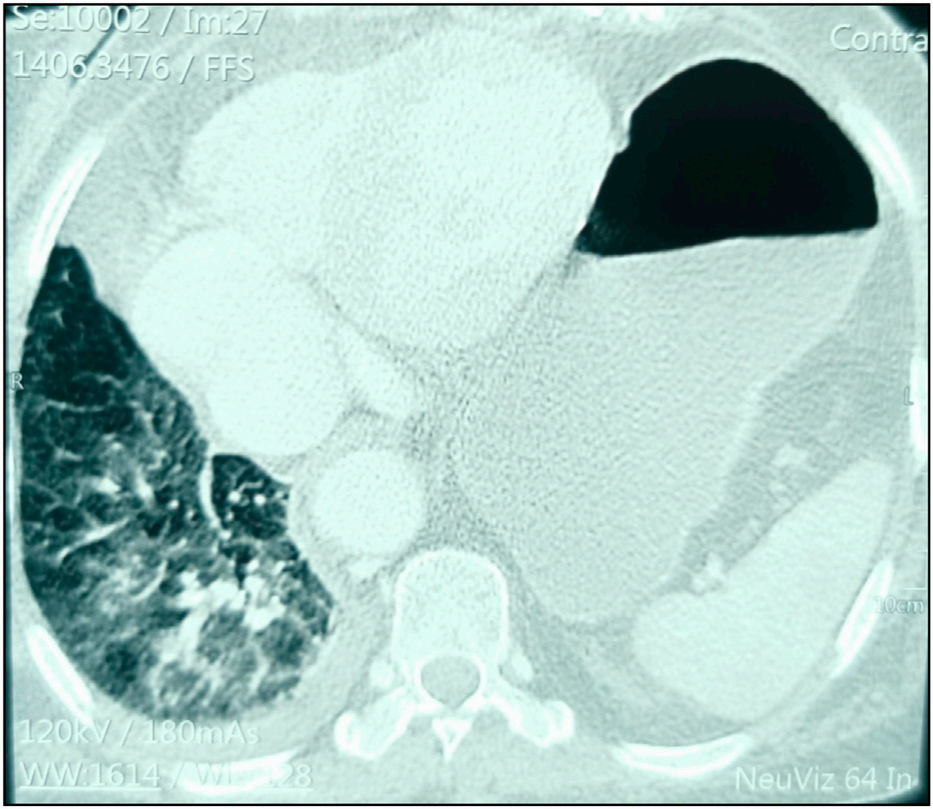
Chest computed tomography showing the spleen and stomach in the left thoracic cavity and condensation of the right lung associated with the mid-pleura.
